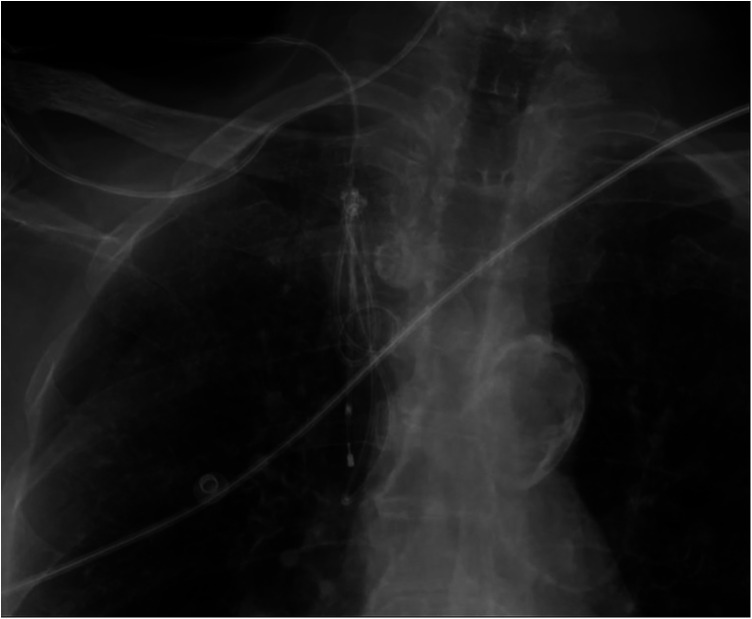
Chest x-ray detail showing the pacing lead loops within the brachiocephalic trunk and superior vena cava, with the knot trapped distal to the introducer and the catheter tip located in the superior vena cava.
